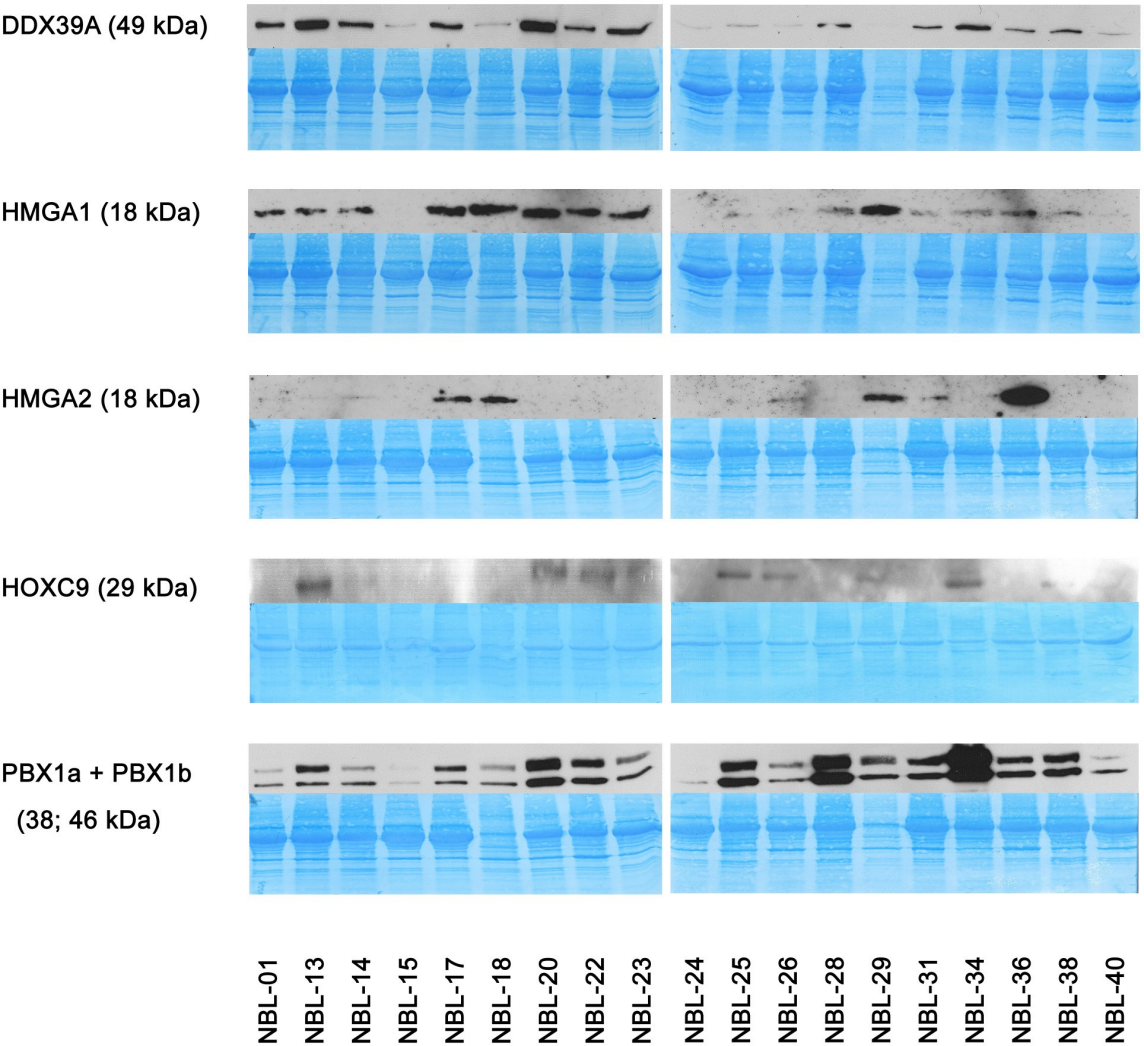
Analysis of endogenous protein levels of putative biomarkers in patient-derived NBL cell lines. Representative immunoblot images captured using X-ray film are shown for each sample. Since looking for reliable loading control protein that would provide satisfactory results throughout all analyzed NBL cell lines was showed to be difficult, loaded amounts of proteins for each sample are documented on the corresponding PVDF membrane scan. As the NBL-30 cell lysate was not obtained in the appropriate quality, this cell line was finally excluded from this screening.
